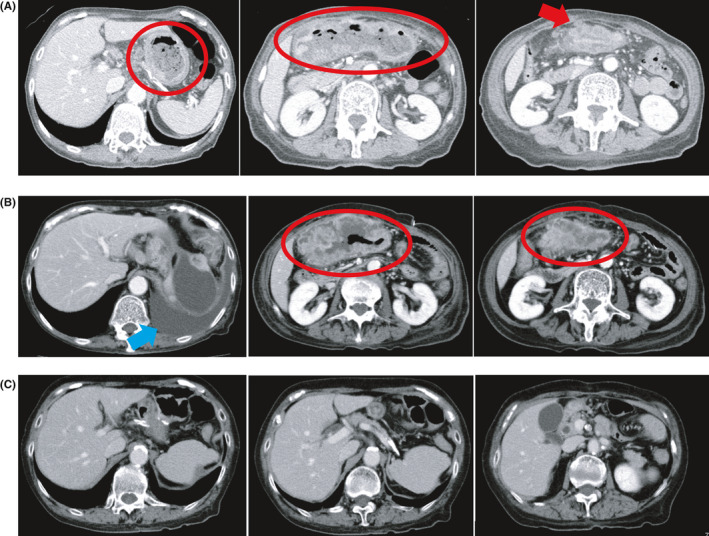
Computed tomography results. (A) At diagnosis: gastric wall thickening (red circle), invasion of the abdominal wall, and regional lymphadenopathy (red arrow). (B) Before nivolumab monotherapy: gastric wall thickening (red circle), invasion of the abdominal wall, regional lymphadenopathy, and pleural effusion (blue arrow). (C) Two years after nivolumab monotherapy: complete response
